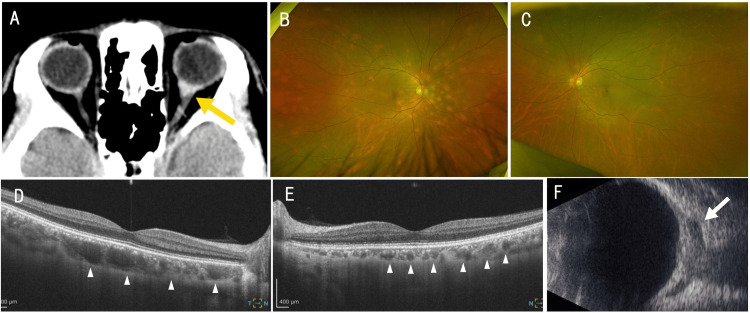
Structural imaging findings of fundus lesions and an optic nerve-associated lesion (A) Non-contrast head computed tomography showing a hyperdense lesion contiguous with the left optic nerve (yellow arrow). (B, C) Ultra-widefield color fundus images of the right eye (B) and the left eye (C), showing multiple deep yellow-orange patchy choroidal lesions. (D, E) Swept-source optical coherence tomography (SS-OCT) images of the right (D) and left (E) eyes show multiple well-defined, hyporeflective, spongiform lesions within the choroidal layer (white arrowheads). Bruch’s membrane, the retinal pigment epithelium, and the neurosensory retina remain intact. (F) B-scan ultrasonography of the left eye also demonstrating a low-echoic lesion continuous with the optic nerve (white arrow).
